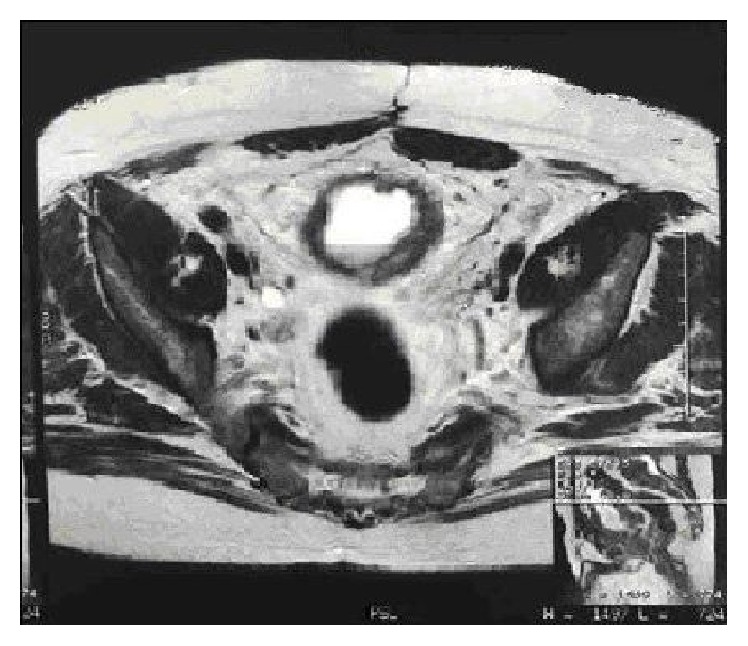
MRI scan section: the MRI scan revealed vesical thickened walls, more evident in the lower third of the bladder, associated with an exophytic lesion.
